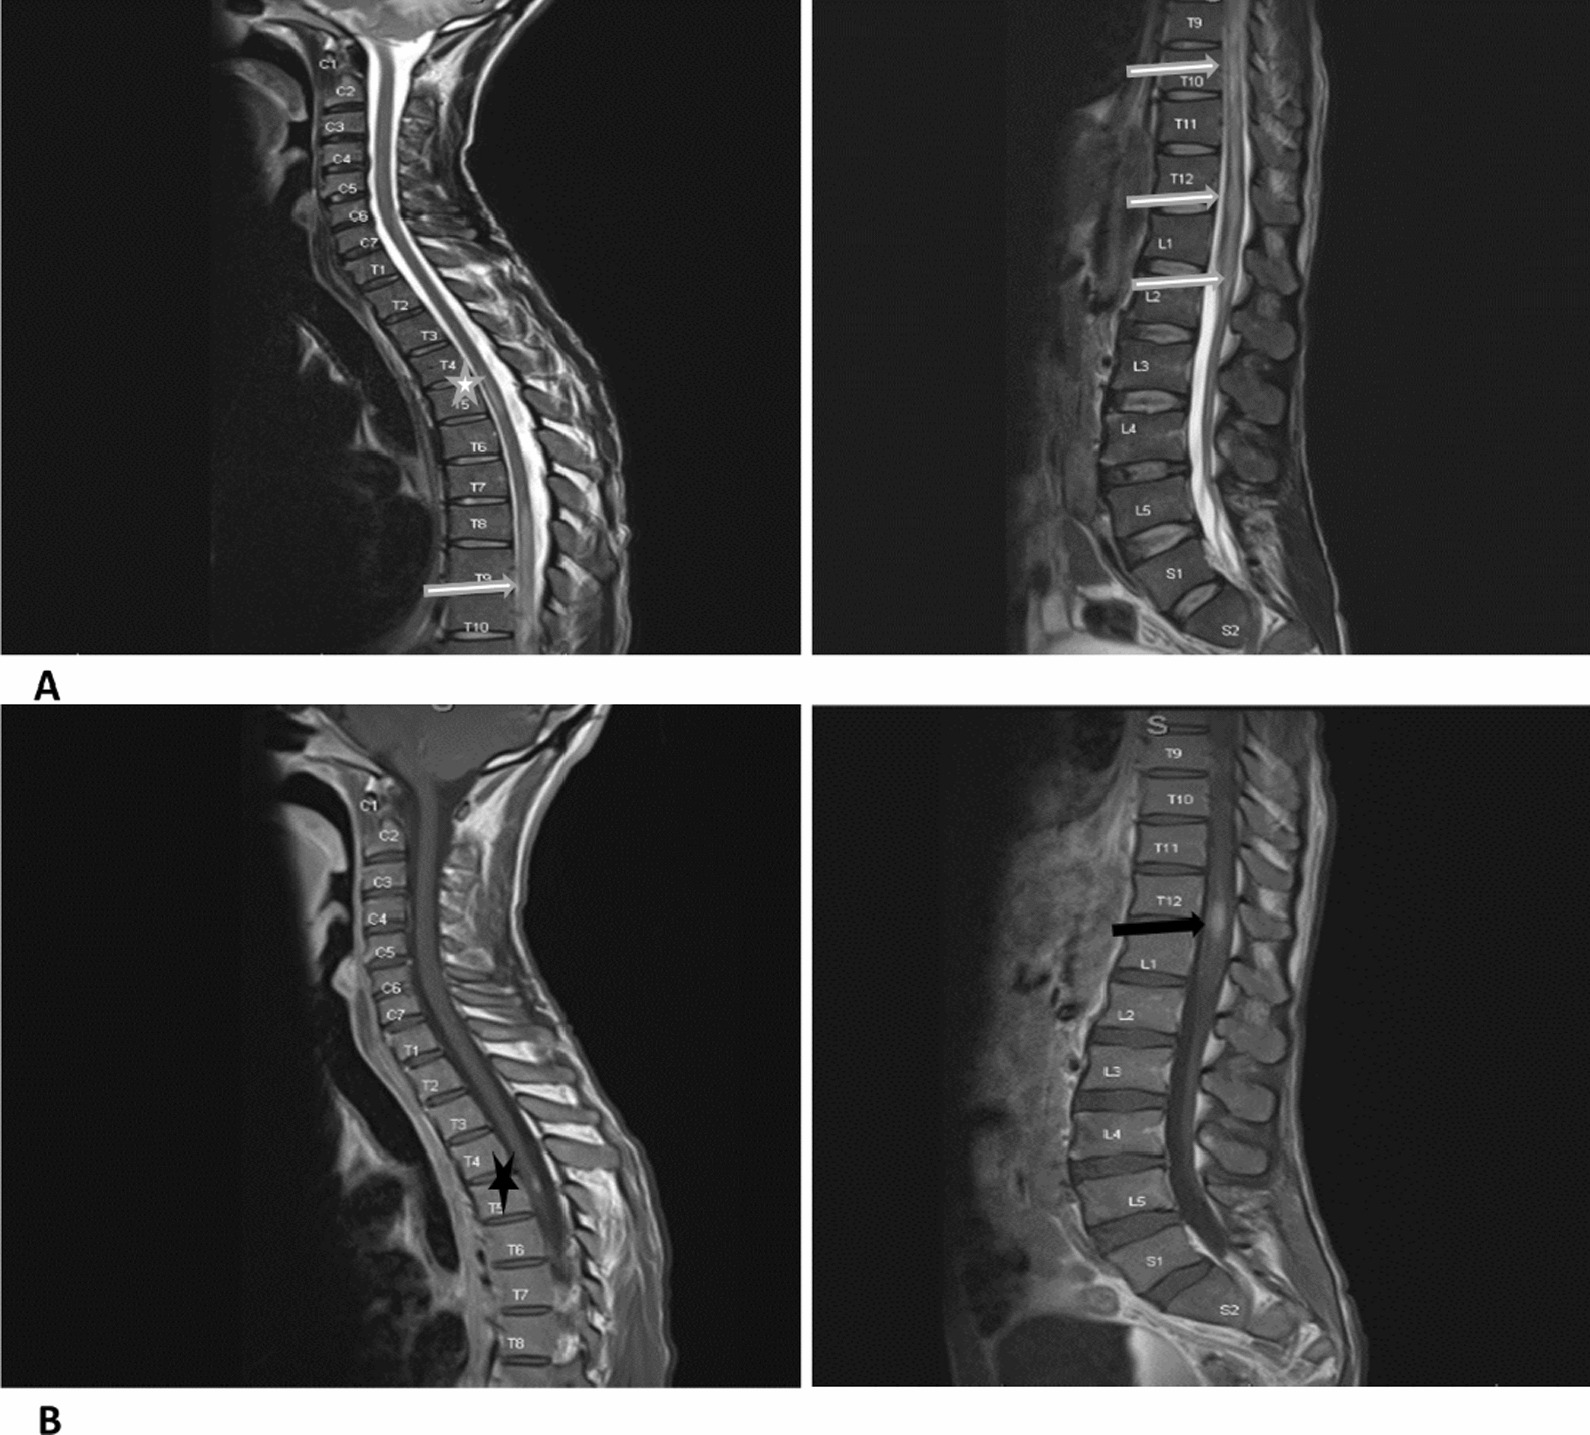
Whole spine magnetic resonance imaging of case 1. Panel A: T2 weighted sagittal images of whole spine demonstrating cord hyperintensities—one short segment at T5 (white star) and a long segment extending from T7 up to and involving the conus medullaris (white horizontal arrows). Panel B: T1 post-contrast sagittal images of whole spine showing small areas of intramedullary enhancement demonstrated at T5 (black star) and T12 (black horizontal arrow)
